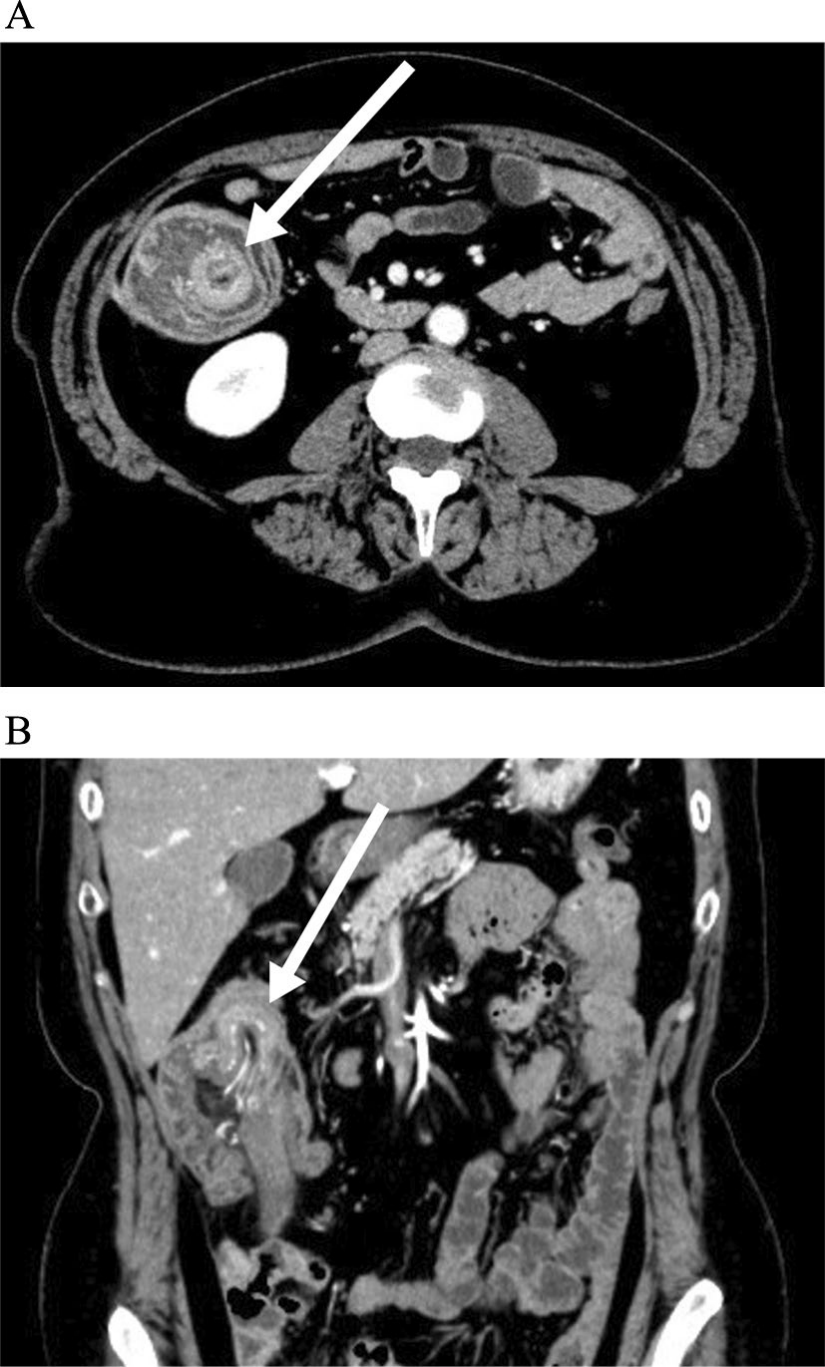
Abdominal computed tomography of abdomen and pelvis with contrast. An ileocolic intussusception is present (arrows) with the cecum polypoid mass lesion telescoping into the ascending colon. A Axial view, B coronal view
